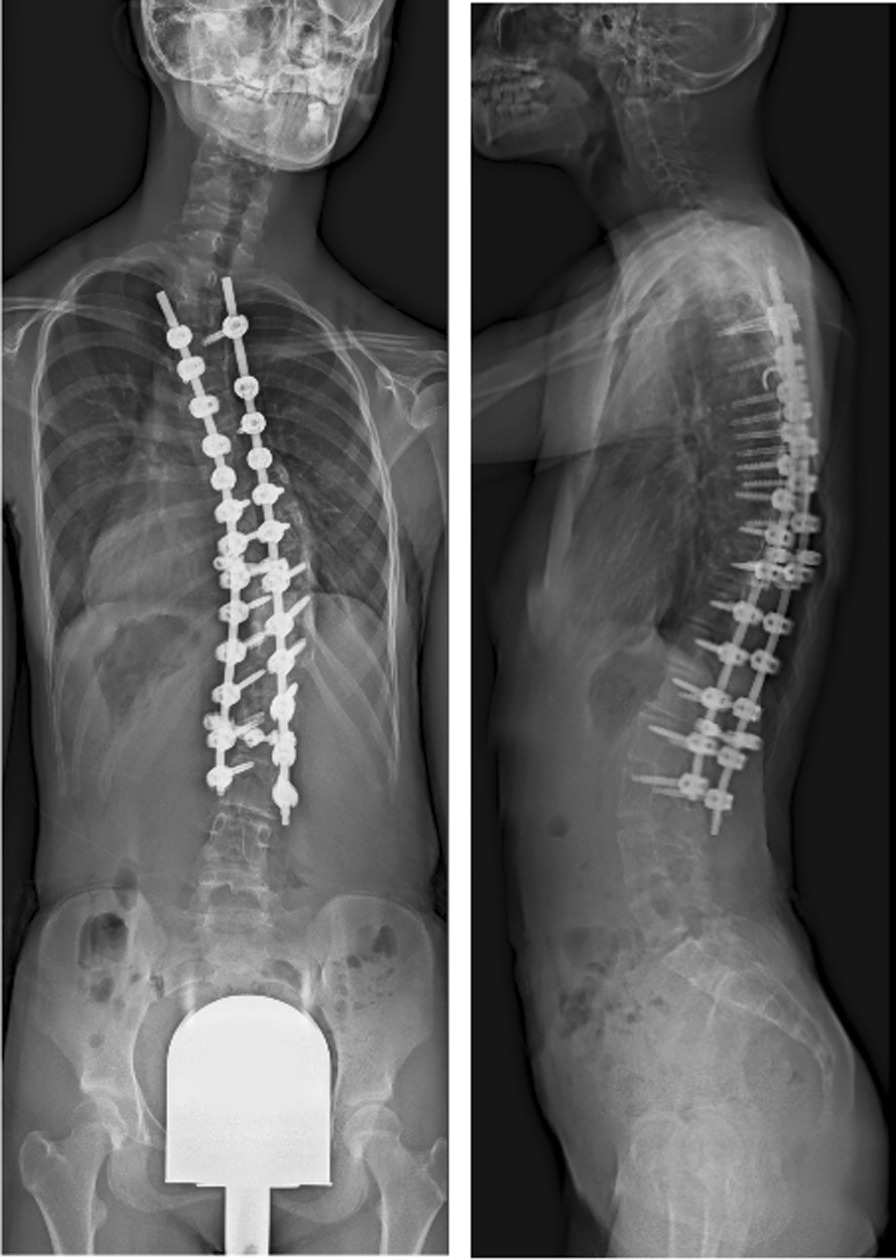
Radiographs of posterior spinal correction surgery at 10 months postoperatively. She underwent posterior spinal correction surgery for severe scoliosis at 12 years of age. Radiographs show a anterior–posterior view and b lateral view
